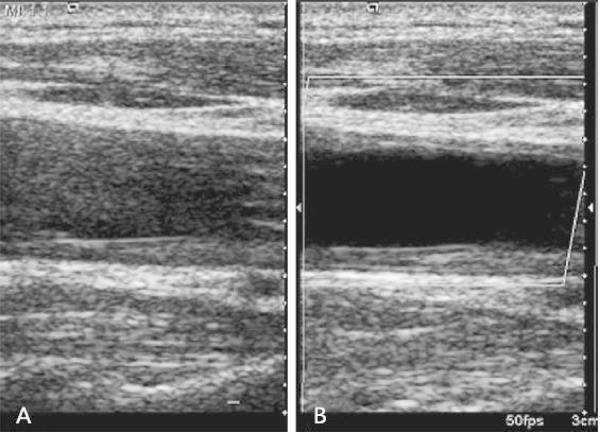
Grayscale 2D ultrasound shows an obscured anterior wall and sound artifacts in the lumen (A), while a VET image shows a clear lumen and a thickened endo-medial tunica of the anterior and posterior wall (B).
